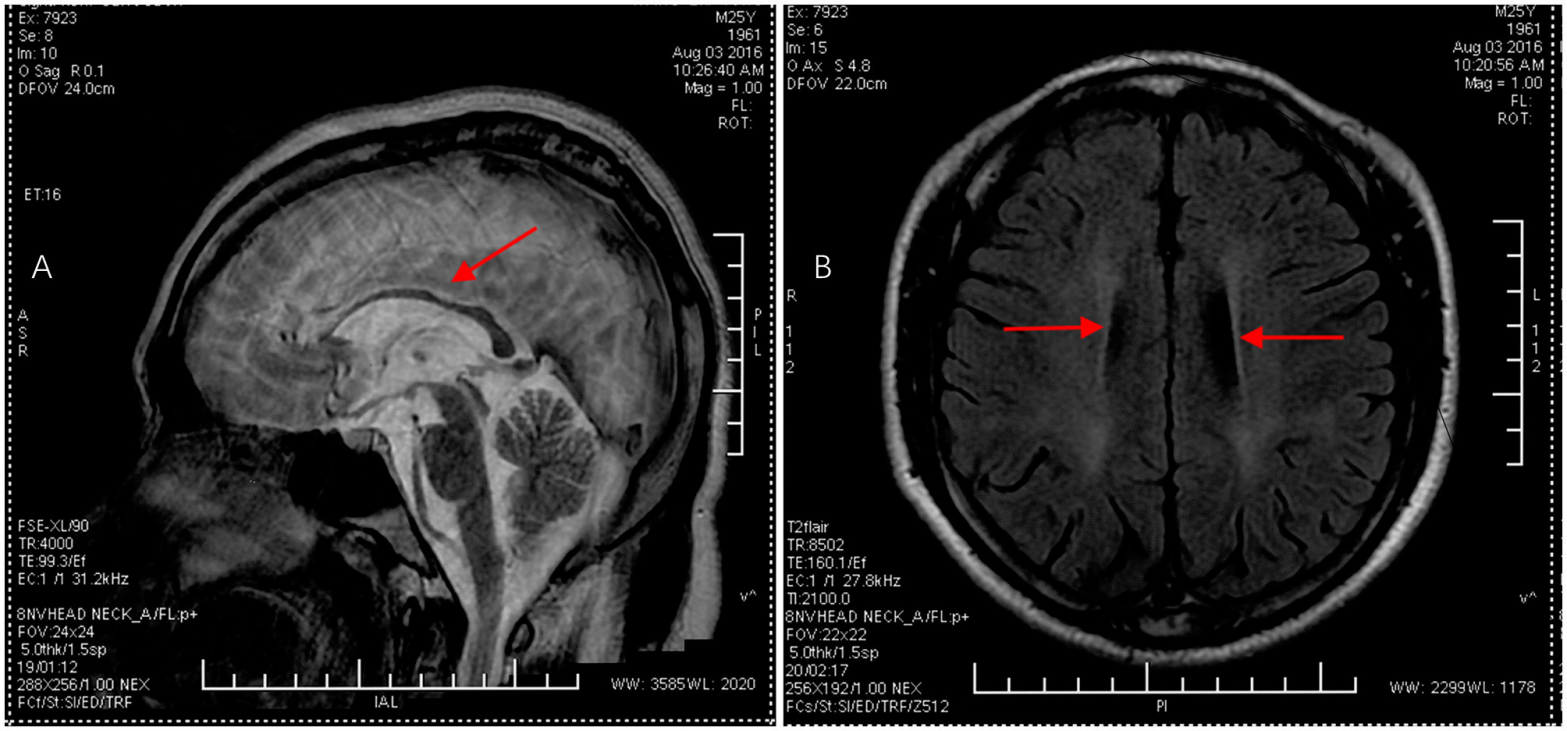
Brain MRI images in the proband. (A) Sagittal T2-weighted image displayed thinning of the corpus callosum, as indicated by the red arrow. (B) Axial T1-weighted image showed symmetrical periventricular white matter lesions, as indicated by the red arrow.
